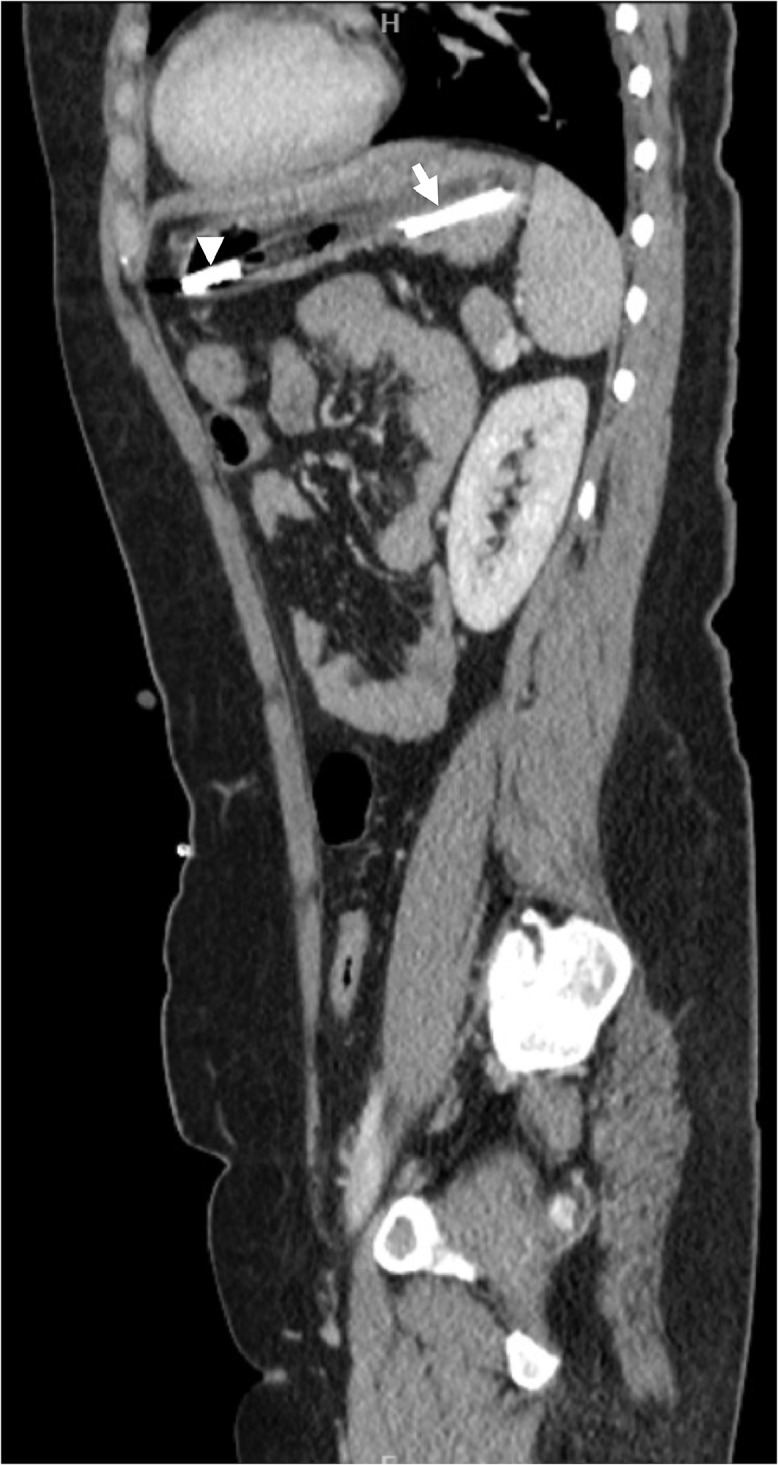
Sagittal CT scan view of the abdomen showing a hair clip (white arrowhead) and a ballpen (white arrow) in the stomach.
